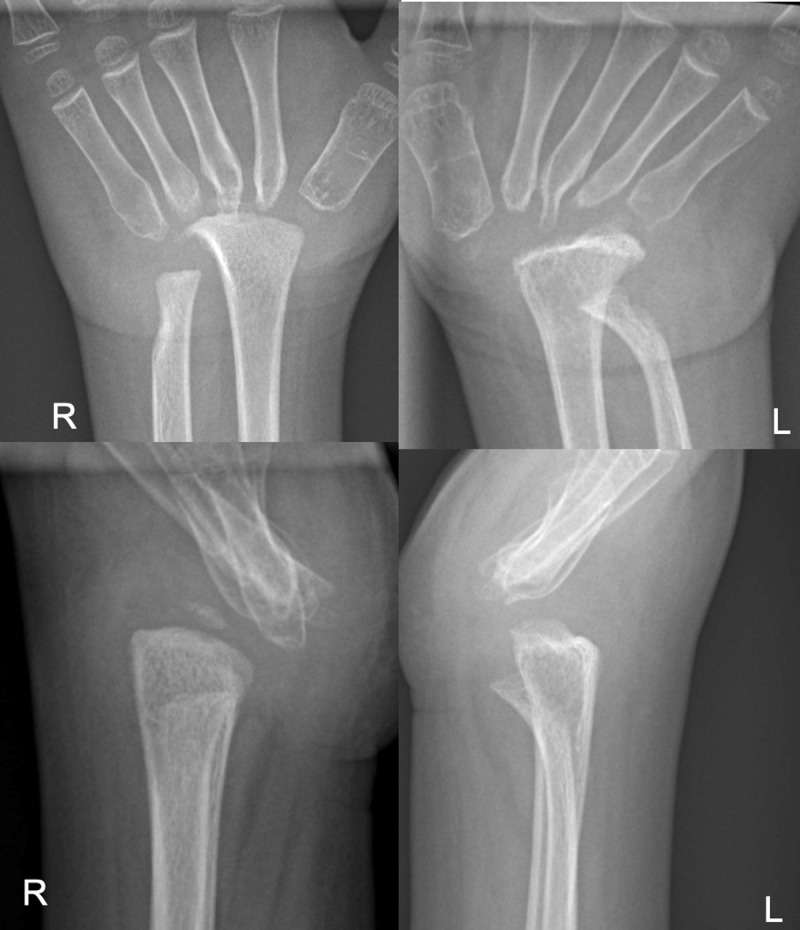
The radiographs of the hands, carpi and distal forearms show a massive osteolysis of the carpal bones bilaterally. The osteolysis also involves the proximal metacarpal bones, which appear tapered and anteriorly subluxated, especially at the right hand. Periarticular soft tissues are swollen with decreased transparancy. The osteolysis of the distal forearm results in radial epiphysis disappearance and radial and ulnar deformity on the left side.
